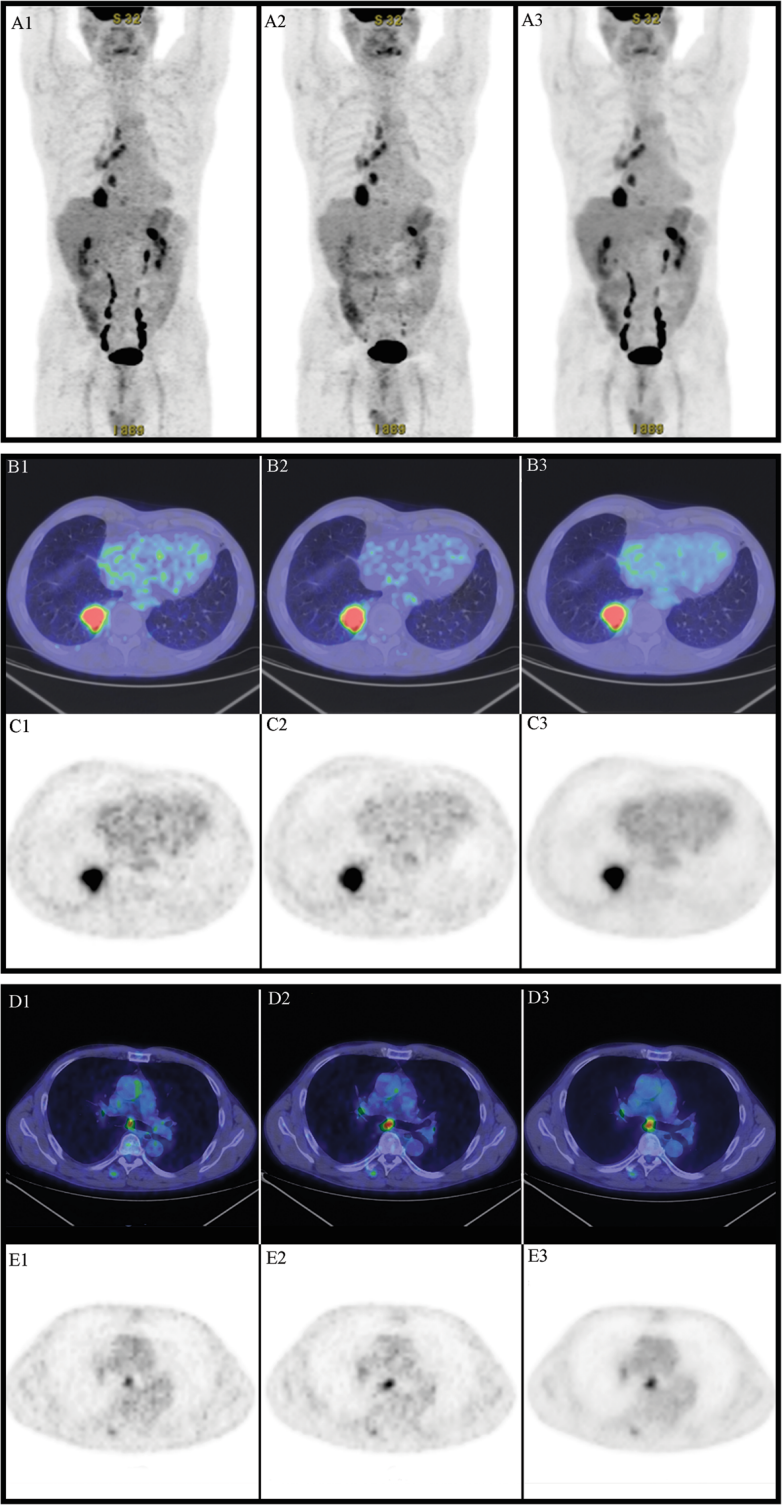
Metabolic characterization of a right lung nodule. Patient injected with 66% dose of 18F-FDG. 1. Scan with reduced number of counts (acquisition with standard time and 66% FDG dose). 2. Native scan: scan with standard number of counts (acquisition with increased time). 3. Processed scan, resulting from the study with reduced counts (1) processed by AI SubtlePET^TM^. a Maximum Intensity Projection (MIP). b, d Fused transaxial positron emission tomography and computed tomography images. c, e Transaxial positron emission tomography images. All images were visualized with AW server station by setting the same parameters (DFOV 57.8 × 35.8 cm, *M*=6.01 g/ml). Lung lesion is easily and appropriately characterized by both native and AI SubtlePET^TM^ images, see MIP (Fig. 5a) and transaxial (Fig. 5b); suspected metastatic lymph nodes are well visualized in both native and AI SubtlePET MIP (Fig. 5a) and transaxial images (Fig. 5c)
